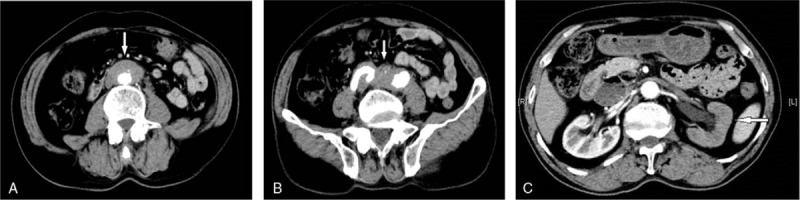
Contrast-enhanced CT of RPF before treatment. (A, B) Axial nonenhanced CT images show an irregular retroperitoneal mass (arrow) which is isoattenuating to muscle. The mass is located anterior and lateral to the lower abdominal aorta and iliac arteries. (C) Left hydronephrosis is secondary to distal encasement of the ureter by the mass. The renal excretion of contrast material was delayed (arrow). CT = computed tomography, RPF = retroperitoneal fibrosis.
